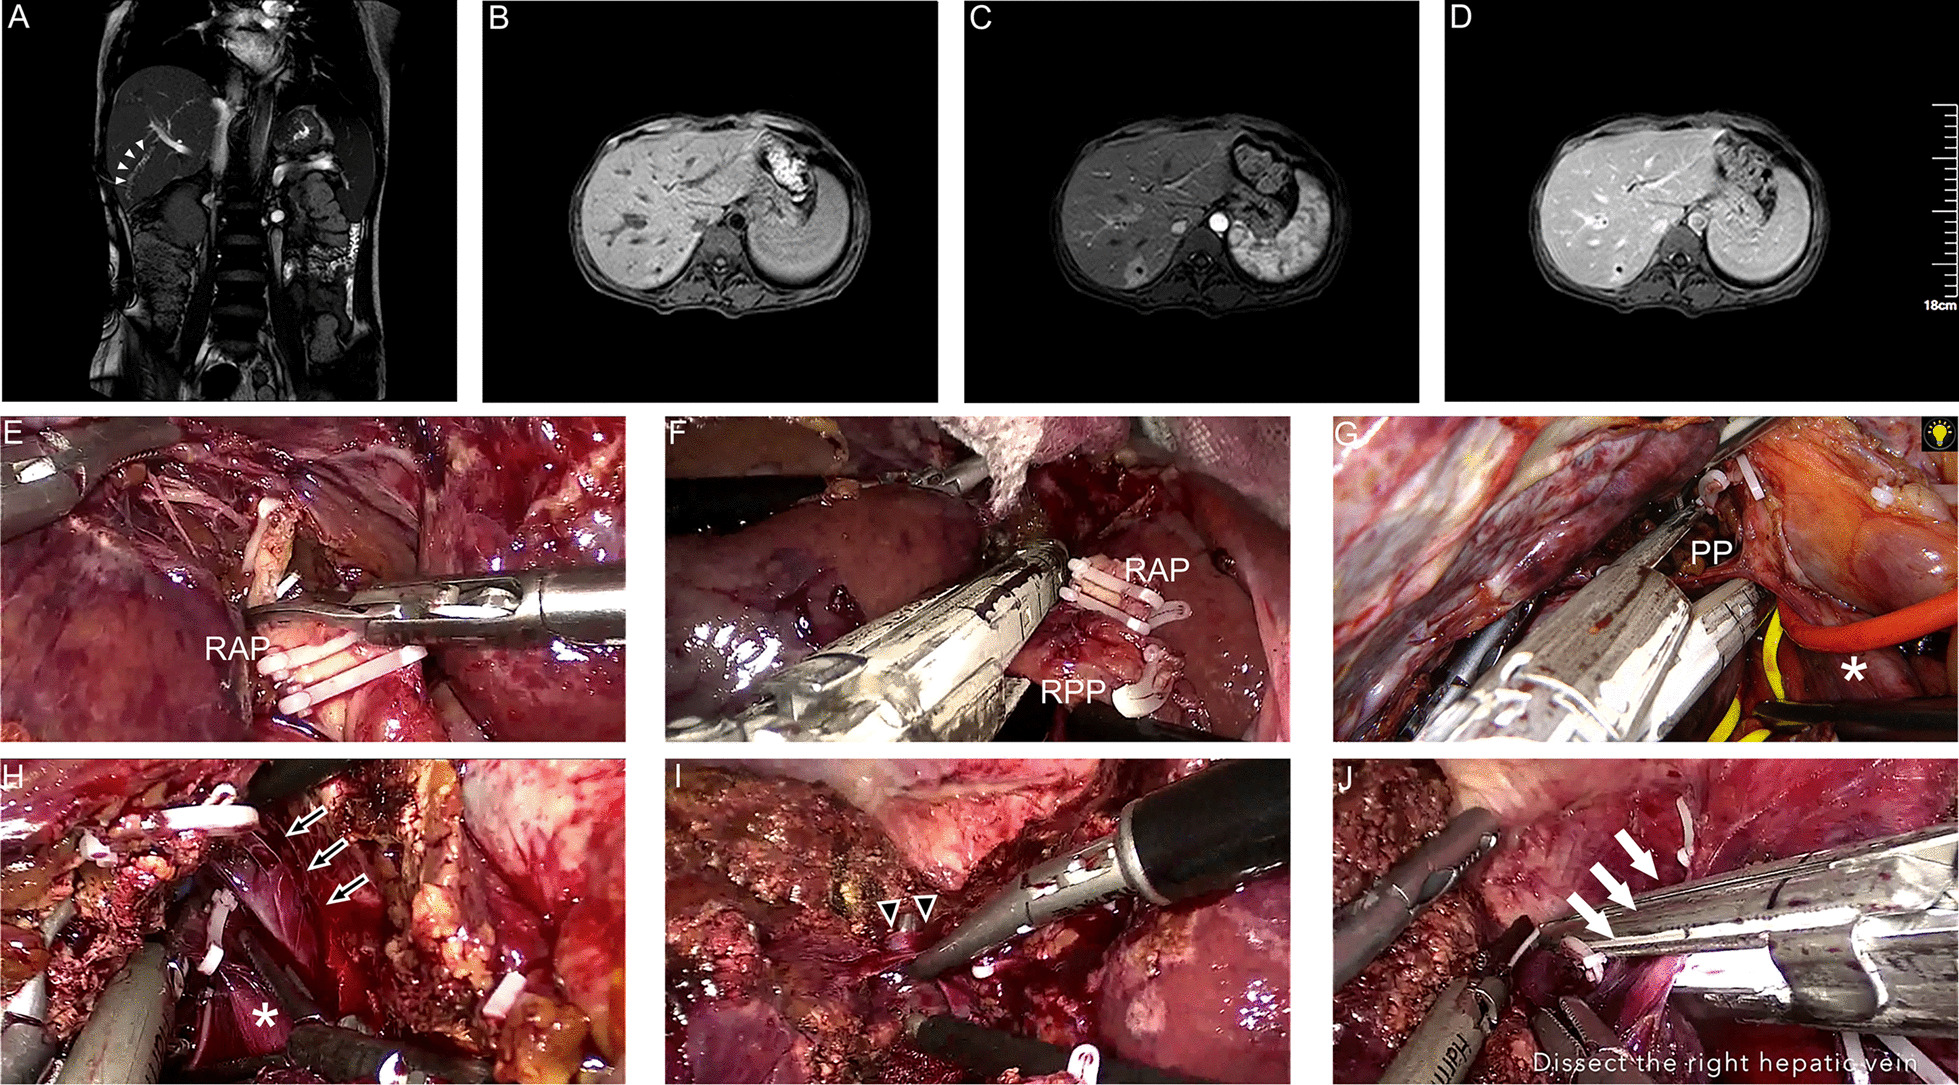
Dorsal approach with Glissonian approach in laparoscopic right hemihepatectomy (LRH). Preoperative MRI showed hepatolithiasis (a–d) and intraoperative key view during procedure (e–j): e to isolate RAP; f to isolate RPP; g to transect PP of the caudate lobe between MHV and IVC through dorsal approach; h to expose MHV and transect liver parenchymal along MHV through dorsal approach; i to isolate S5HV through ventral approach; j to dissect RHV. White arrowheads, hepatolithiasis; white asterisk, IVC; black arrows with white edge, MHV; black arrowheads with white edge, S5HV; white arrows, RHV
